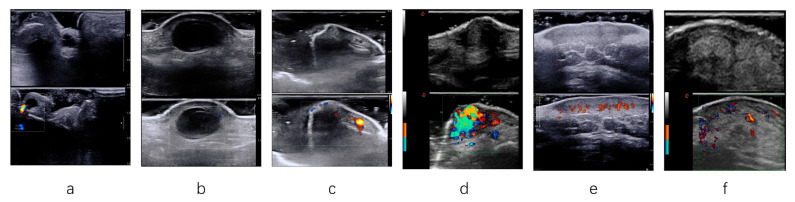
Figures (a–f) show the ultrasound image features of digital mucous cyst, thecal cyst, wart, angioneoplasm, malignant melanoma, and cutaneous squamous cell carcinoma in sequence. (a) B-mode sonogram showing an echoless mass with regular margins. CDFI sonogram showing no vascularity in the lesion. (b) B-mode sonogram showing an echoless mass with regular margins and adjacent to the thecal cyst. CDFI sonogram showing no vascularity in the lesion. (c) B-mode sonogram showing a hypo-echoic flat mass with irregular margins; there is sound attenuation behind the lesion. CDFI sonogram showing no vascularity in the lesion. (d) B-mode sonogram showing a hypo-echoic mass. CDFI sonogram showing extremely rich increased vascularity in the lesion. Transient blood flow signal enhancement is visible in the compressed lesion. (e) B-mode sonogram showing a hypo-echoic mass. The lesion grows downward from its base, exhibiting an invasive behavior. CDFI sonogram showing increased vascularity in the tissue. (f) B-mode sonogram showing an iso-echoic mass with irregular and blurred margins. Excessive keratinization of the lesion surface. CDFI sonogram showing increased vascularity in the mass.
